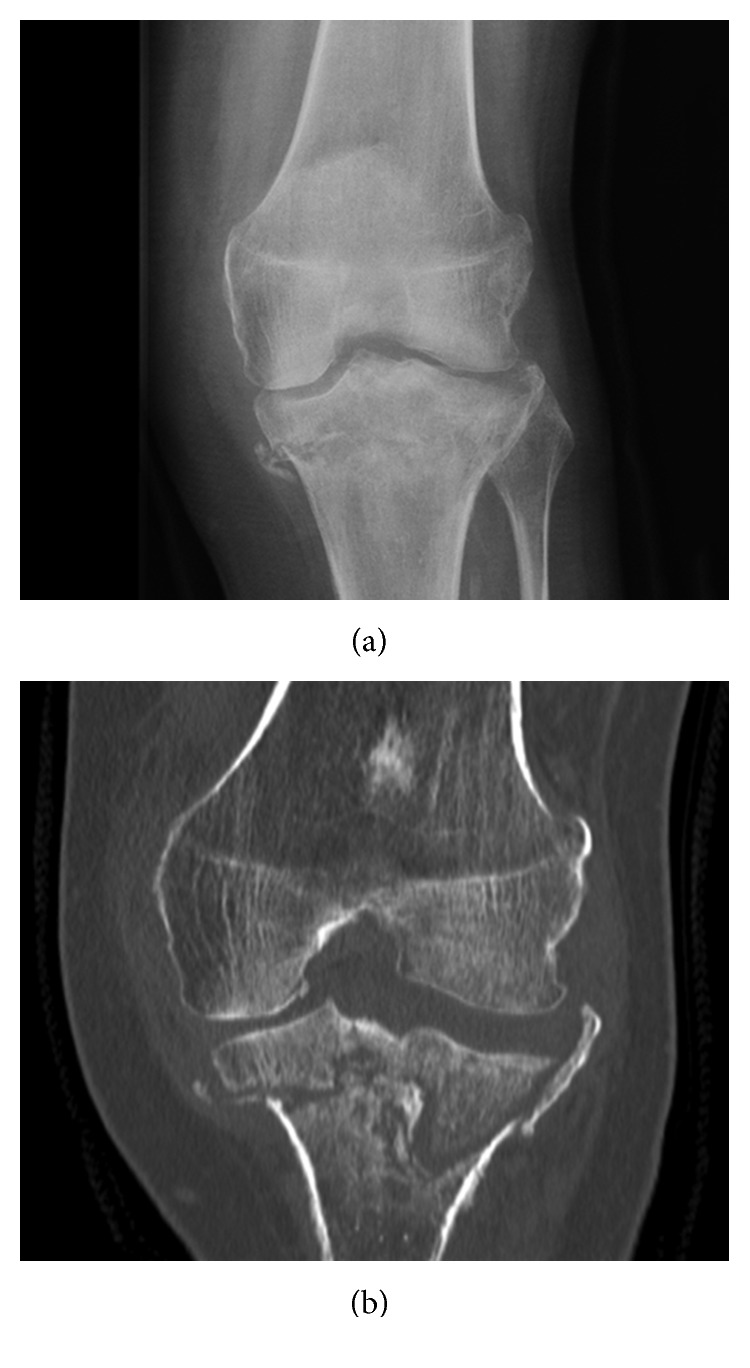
Delayed healing. (a) AP radiograph and (b) coronal CT scan before Exogen treatment.
